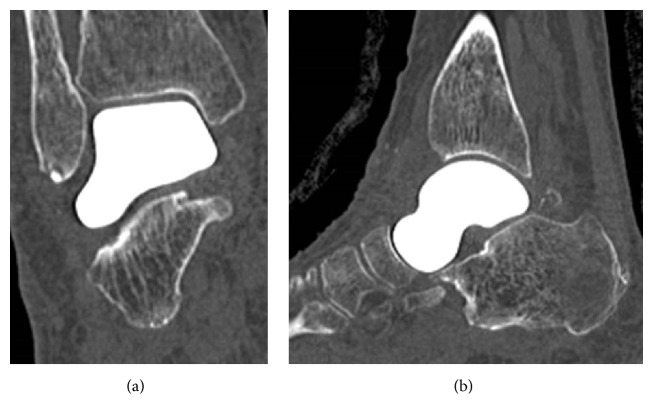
Postoperative CT scans showing good alignment of the prosthesis and surrounding bones in coronal (a) and sagittal (b) views at 1 year and 6 months after surgery. There is no osteolytic change in the surrounding bones or sinking of the prosthesis.
